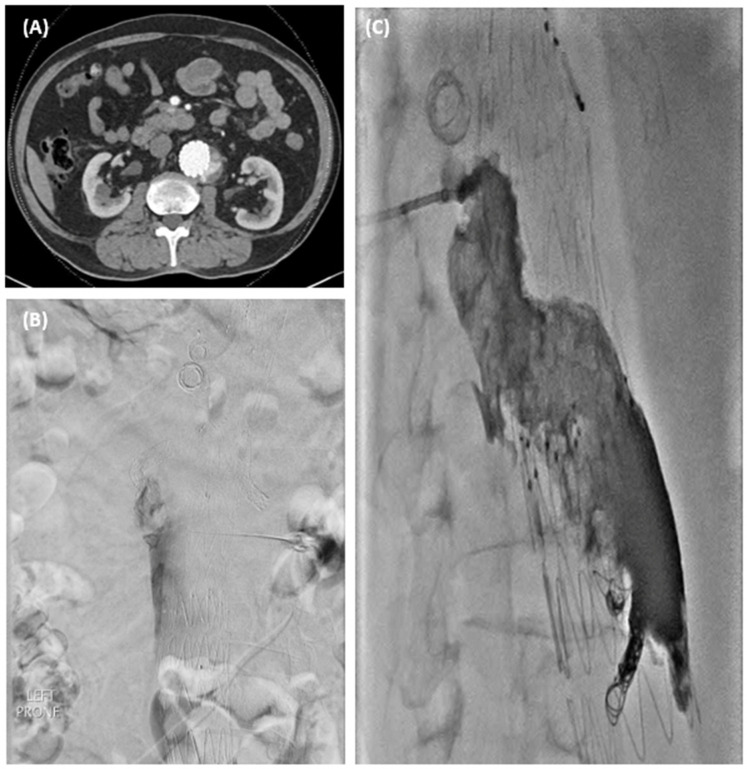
(A) CT showing Type 2 endoleak with increasing sac on surveillance. (B) Translumbar sac puncture performed under CT guidance with saccogram performed. (C) Coil embolisation with Concerto coils of IMA and rest of endoleak cavity filled with glue.
